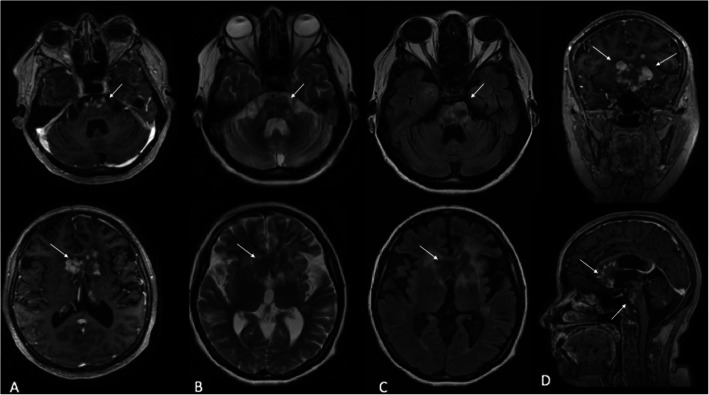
Follow up brain MRI performed 15 months after the first MRI showed in Fig. 1, in corresponding levels and same sequences, showing significant regression of vasogenic edema and partial decrease in lesion size (white arrows) after treatment. a Axial T1 post contrast at the level of the pons and basal ganglia. b Axial T2 images. c Axial FLAIR images. d Coronal (on top) and sagittal T1 (bottom) post contrast images
